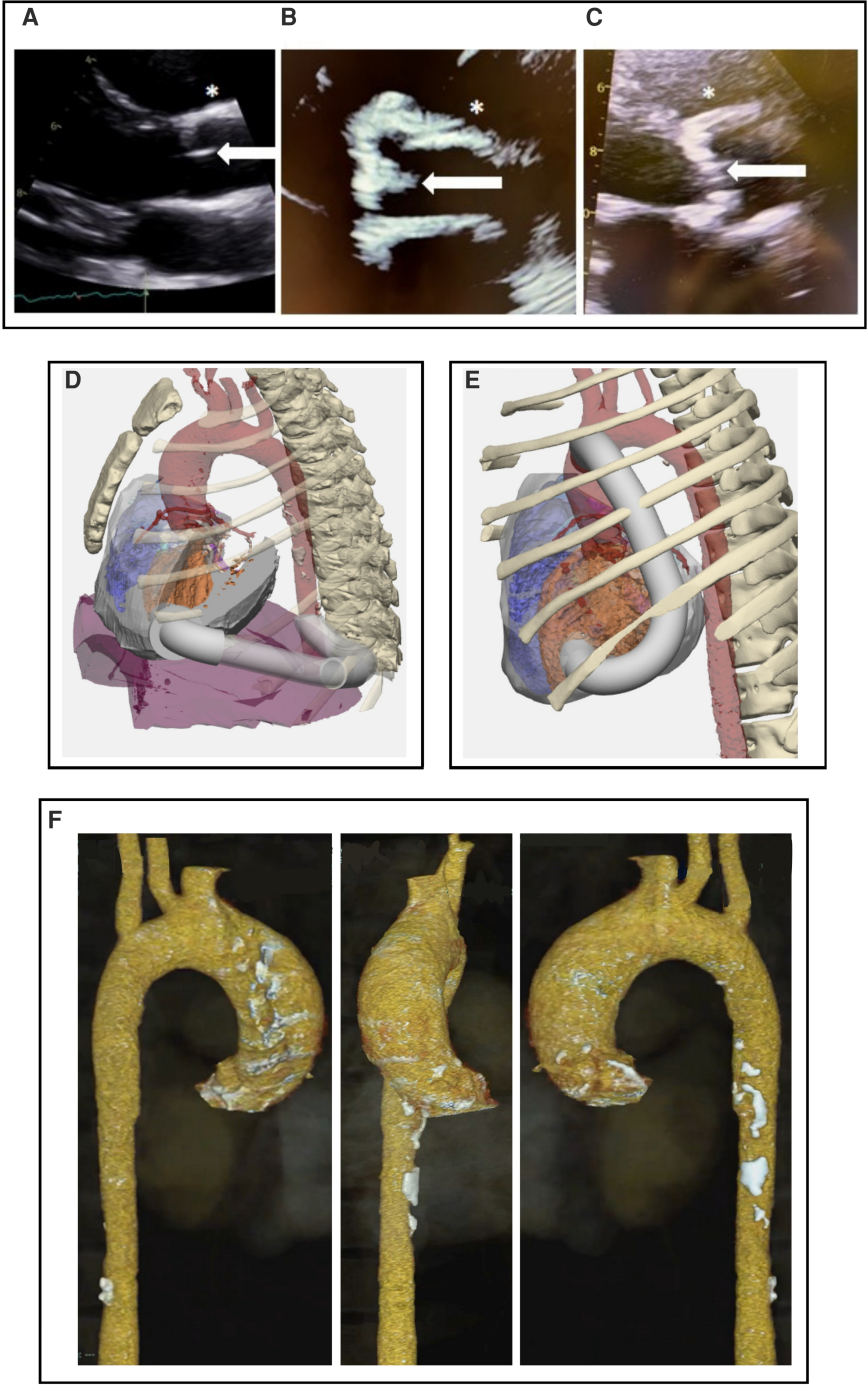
(A–C) Parasternal long axis echocardiographic view at the level of the aortic valve. (A) 19 y.o healthy control. (B) 22 y.o with HGPS and advanced aortic stenosis. (C) 72 y.o. with calcific aortic stenosis, demonstating calcific appearance in both aging and HGPS which is unlike the congential aortic stenosis seen in children. Note bright, diffusely thickened aortic valve (white arrows) and aortic wall (*) in (B) and (C) compared to (A). (D,E) 3-dimensional CT with AAC modeling performed prior to surgical intervention for cases #3 and #4. Design with conduit (white) origin at cardiac apex and insertion into (D) descending aorta and (E) ascending aorta. Orange is left ventricle; red is aorta. (F) CT 3D reconstruction of case #3 demonstrating calcification throughout the aortic arch and ascending aorta.
